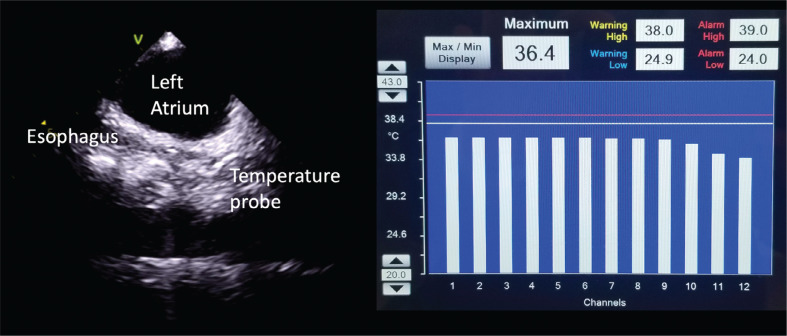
Fluoroless visualization of a multipoint, sinusoidal esophageal temperature probe (CIRCA S-CATH) using intracardiac echocardiography. The temperature spread can confirm the proper insertion depth; the esophageal temperature is lower on the proximal electrodes (10–12) closer to the trachea above the left atrium.
